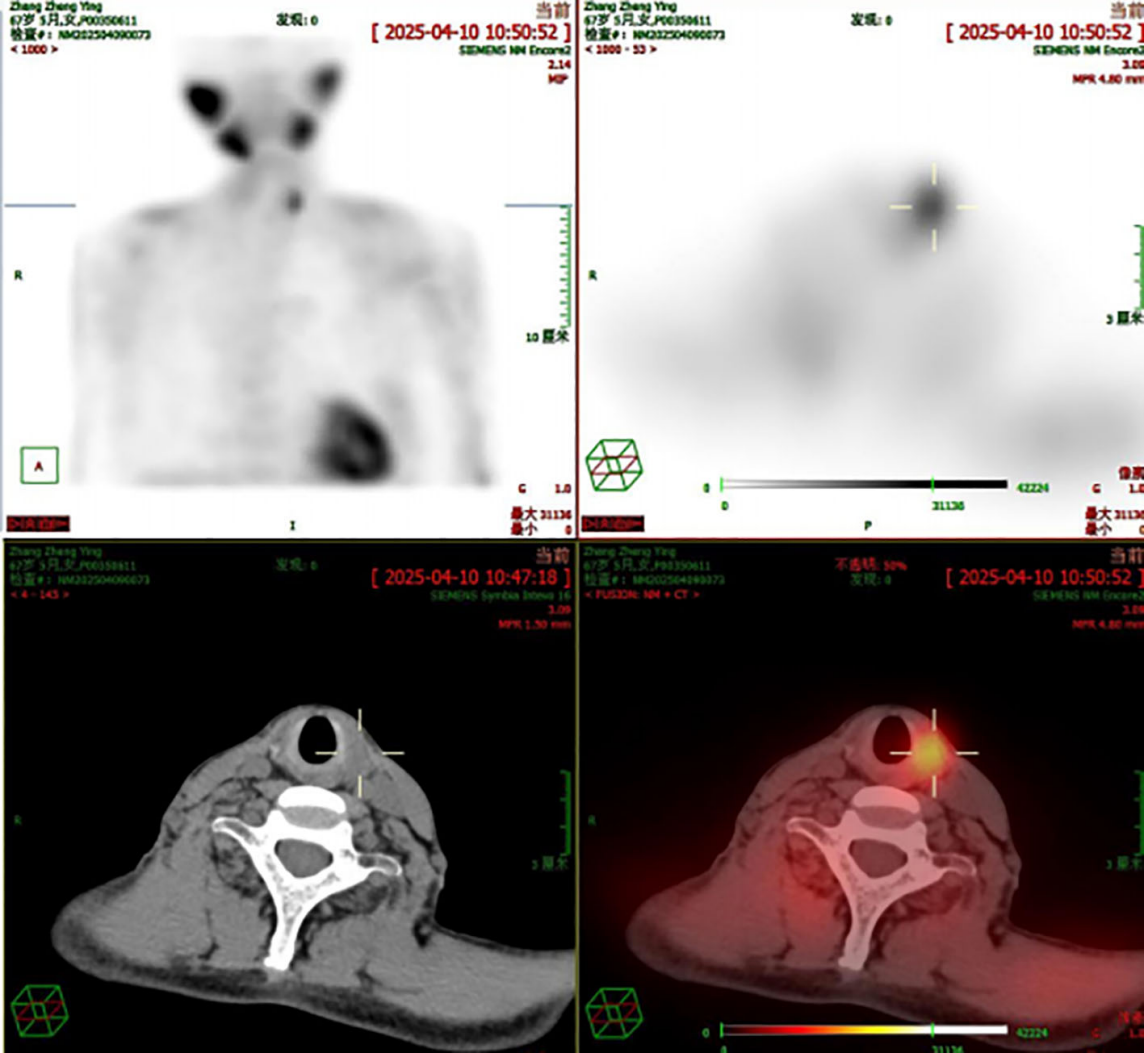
SPECT scintigraphy of the patient.
